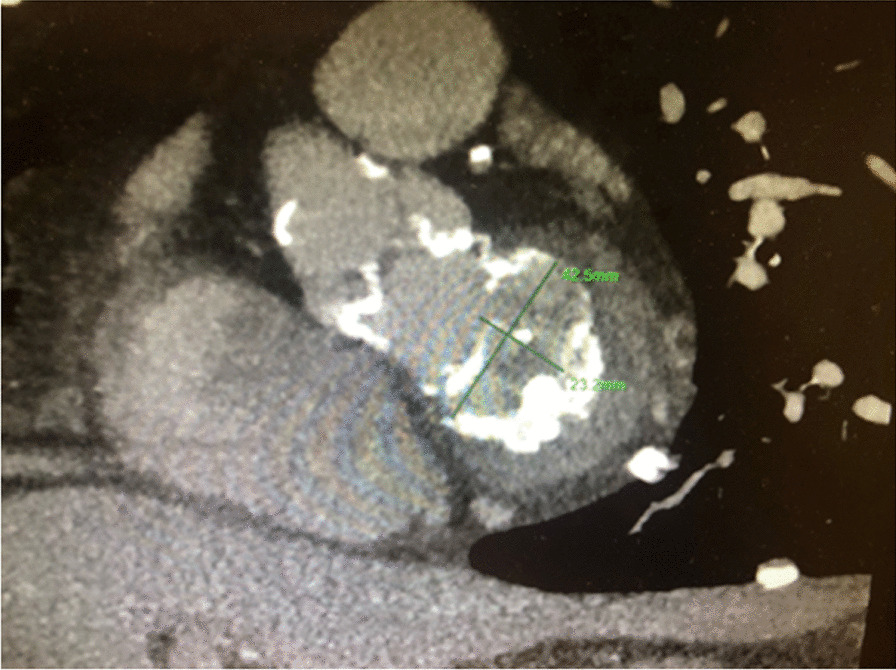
Cardiac-gated CT demonstrating severe mitral annular calcification with annular dimensions of 42.5 mm × 23.2 mm
